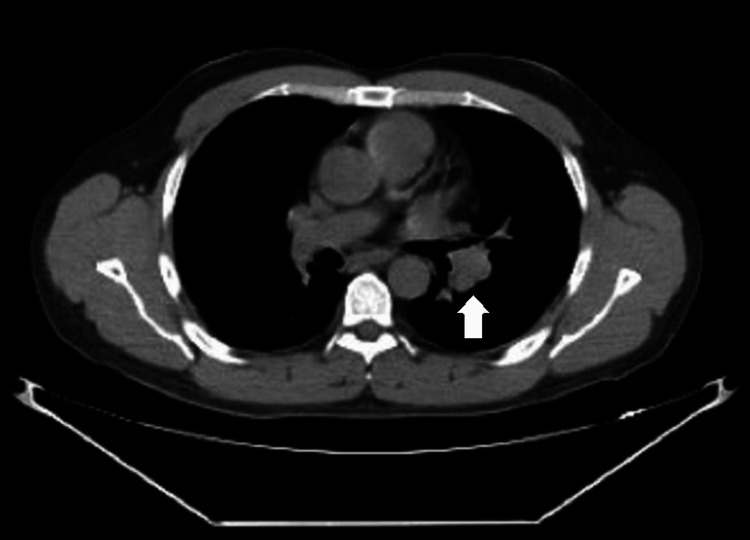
CT imaging of the left hilar mass, visually indicated by a distinct white arrow.
